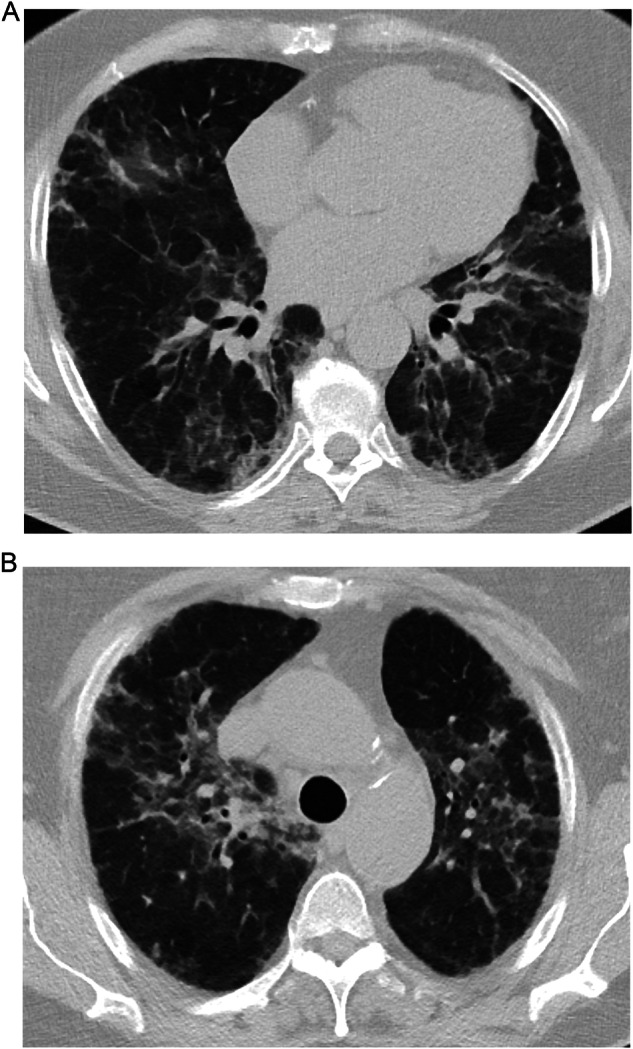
A, B CT scans of a patient with systemic anticancer therapy-induced interstitial lung disease showing the non-specific interstitial pneumonia pattern, diagnosed by multidisciplinary team consensus
